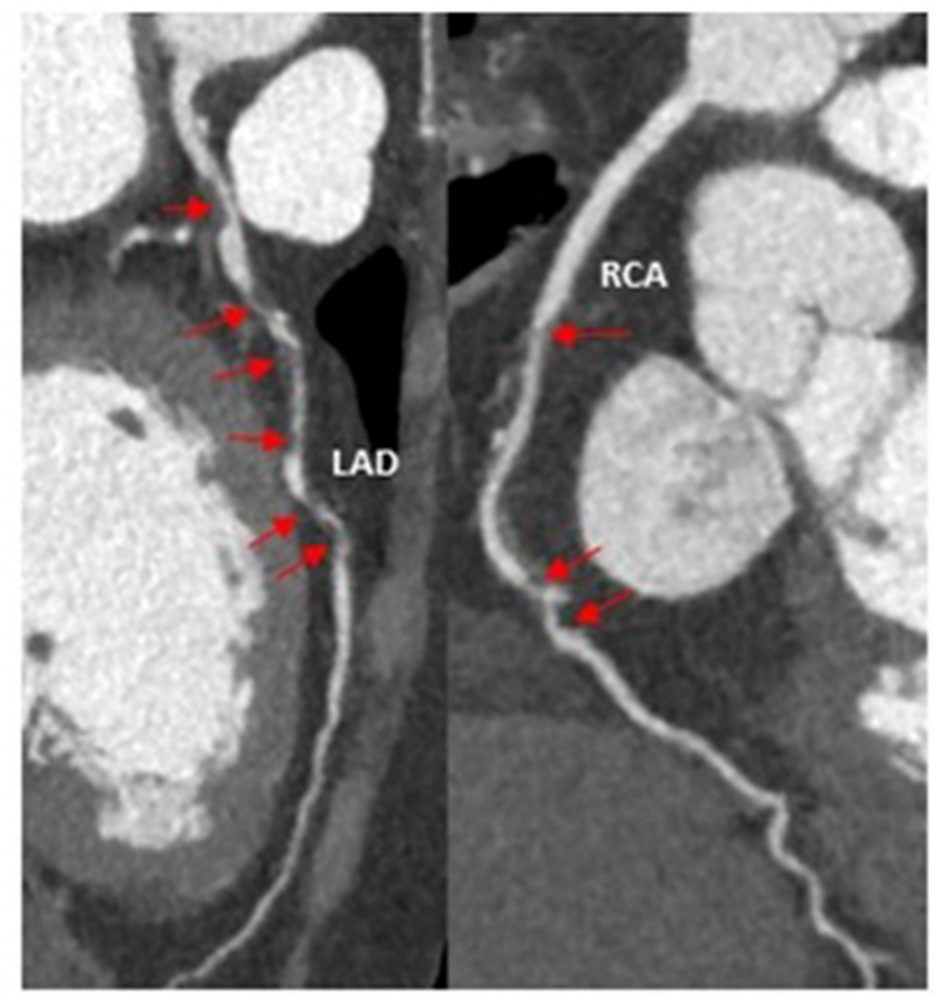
Coronary computed tomography angiography indicated critical stenosis (red arrows) of right coronary artery and left anterior descending coronary artery.
